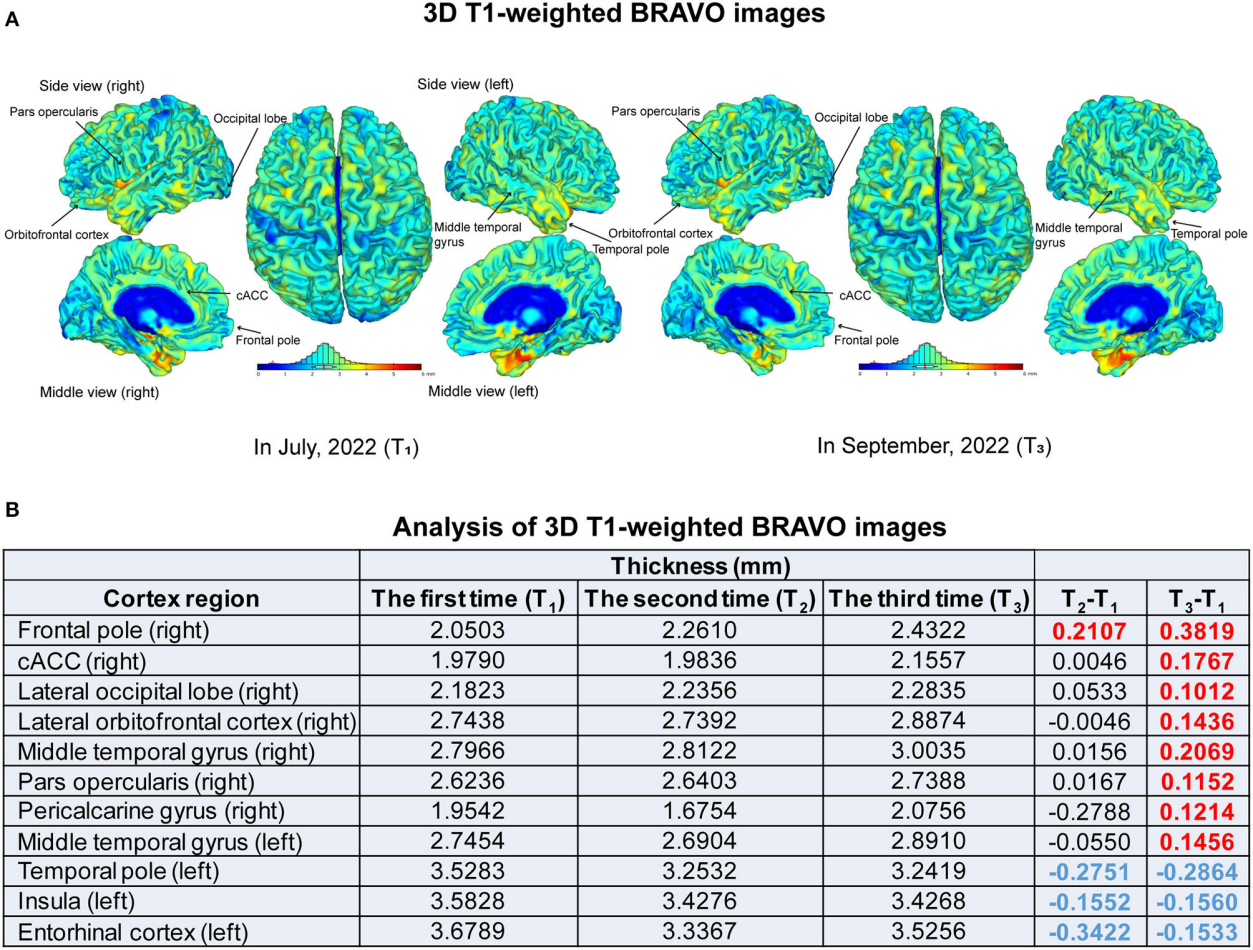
High-resolution 3D T1-weighted BRAVO images. (A) 3D BRAVO images before treatments (T_1_) and at the end of 2-month treatments (T_3_) of mirror therapy and sacral plexus magnetic stimulation. Different colors demonstrate different thicknesses, as shown with the color bar. The black arrows indicate regions with changed thickness. (B) Analysis of 3D T1-weighted BRAVO images obtained before treatment (T_1_) in July, at the end of 1-month treatment (T_2_) in August, and at the end of 2-month treatment (T_3_) in September 2022.
